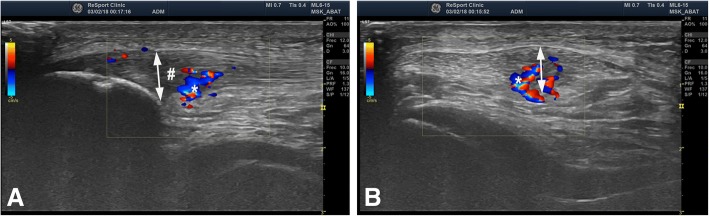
US+Doppler picture (Longitudinal (a) and transversal (b) view) from a patient suffering from proximal patellar tendinopathy-Jumper’s knee, showing a thickened patellar tendon (doble head-arrow) with structural changes and hypo-echoic regions (#) together with high blood flow (*) inside and outside the dorsal side of the tendon
